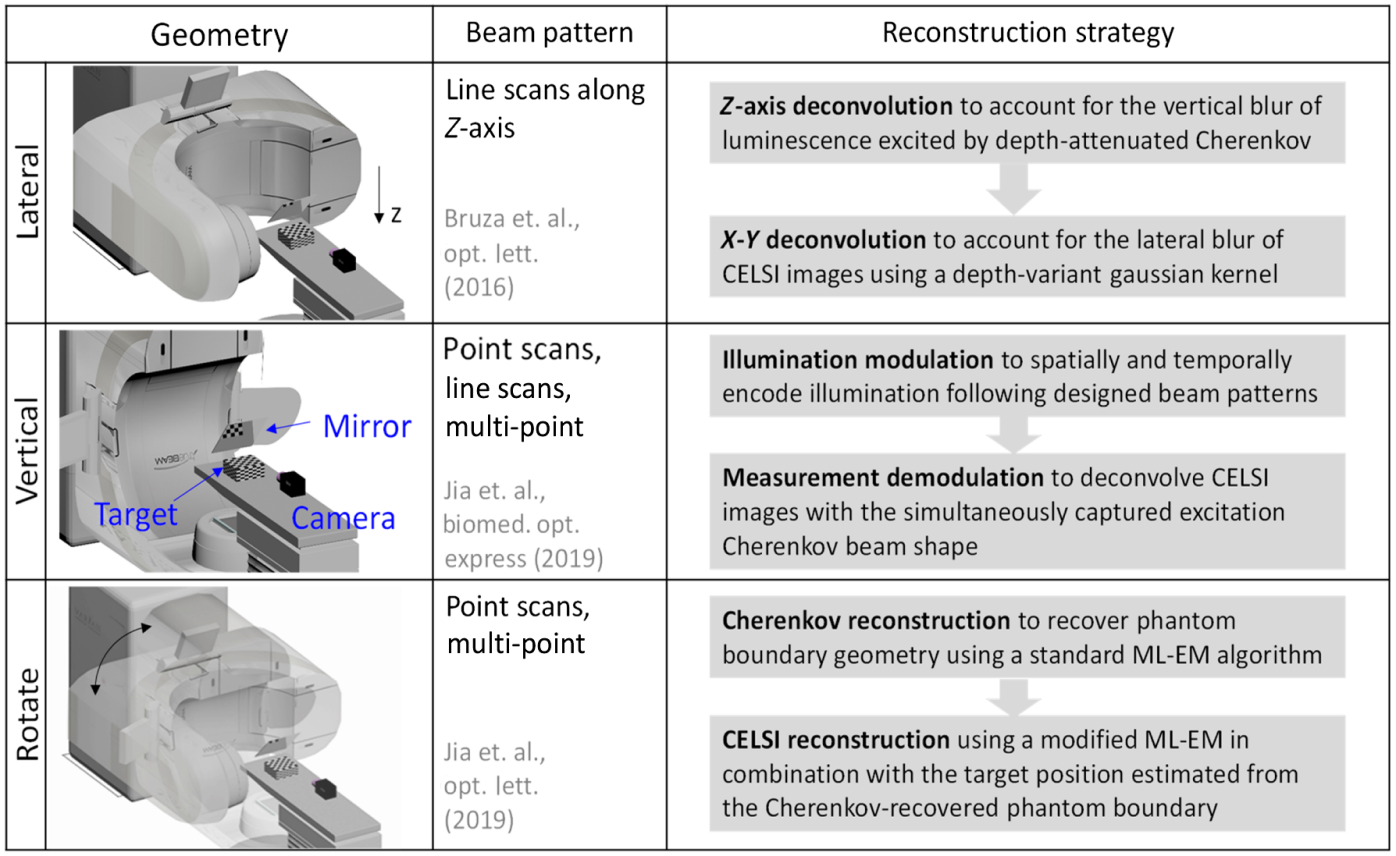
Cherenkov radioluminescence measurement geometries based on Linac MLC delivery and corresponding non-model-based image reconstructions. Laterally scanned imaging (top) sends sheet-shaped x-ray beams to measure local luminescence emission that is then deconvolved with the kernels derived from a diffusion approximation. Vertically scanned imaging (middle) spatially and temporally modulates the Cherenkov illumination following specific beam patterns and then demodulation with the simultaneously acquired excitation Cherenkov beam shapes is possible. Like the imaging technique used in CT (bottom), CELSI images were acquired by rotating the Linac gantry to yield a sinogram, which was then used with FBP of the data for image reconstruction. In all experiments, a plane mirror was always used to avoid direct exposure of the imaging camera to the radiation beam.
